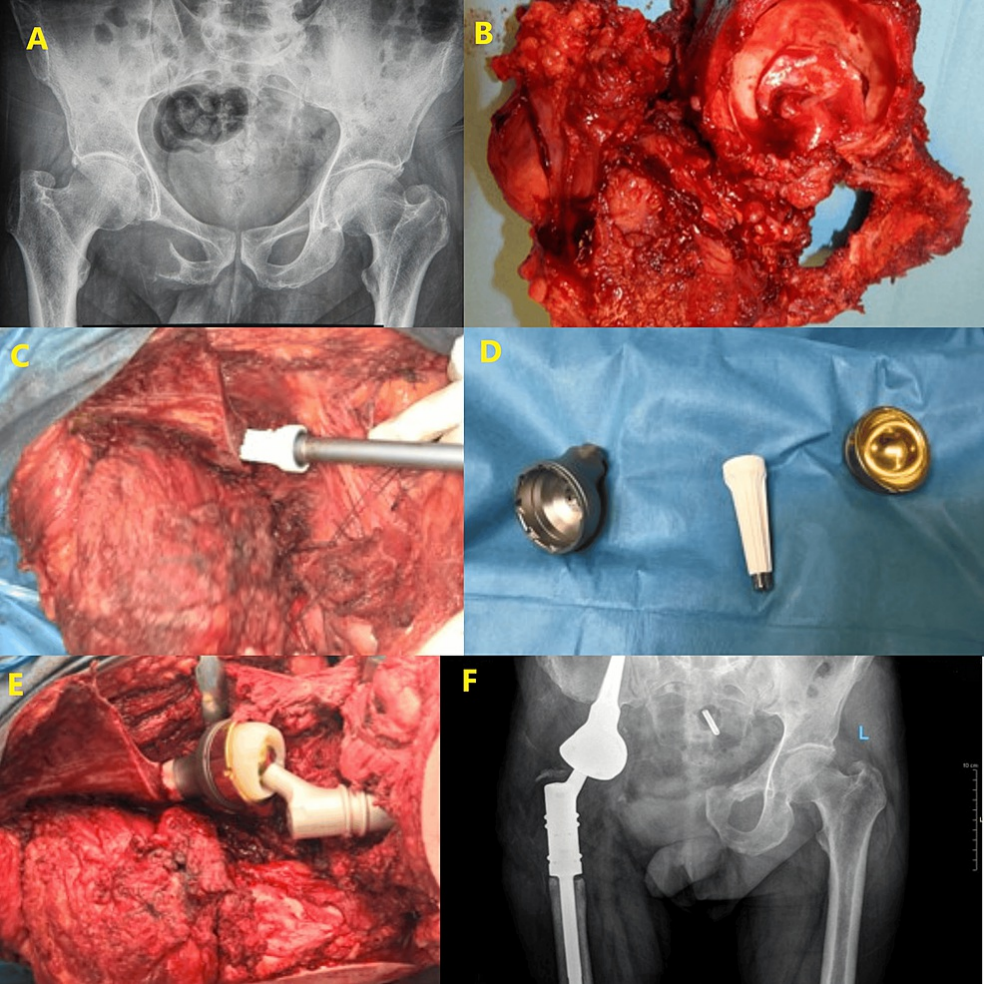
A: The pre-operative radiograph of a 50‐year‐old male patient with chondrosarcoma of the pelvis. B: The patient underwent a wide resection. C: Bone preparation. D: LUMiC endoprosthesis. E: A proximal femur replacement with a LUMiC endoprosthetic device and trevira tube were placed. F: One-year follow-up with radiograph.
